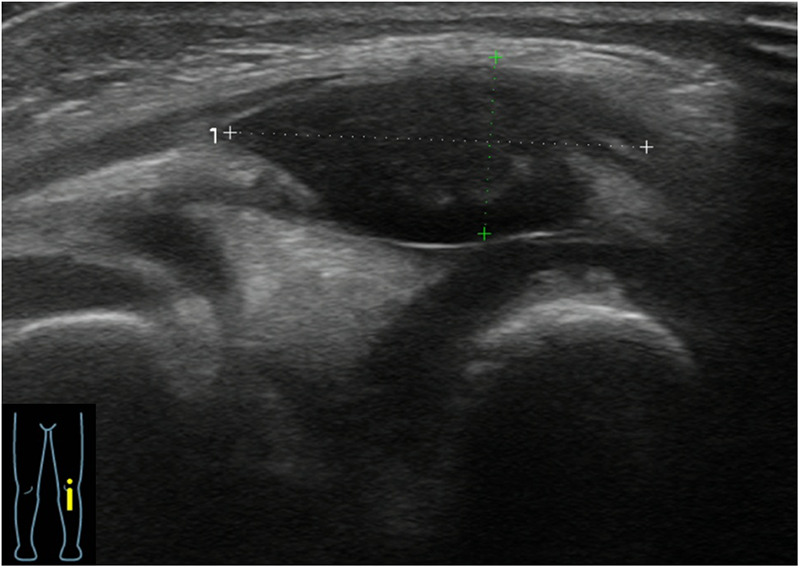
Ultrasonography of the left knee showing a hypoechoic fluid collection with internal and flocculent echoes.
